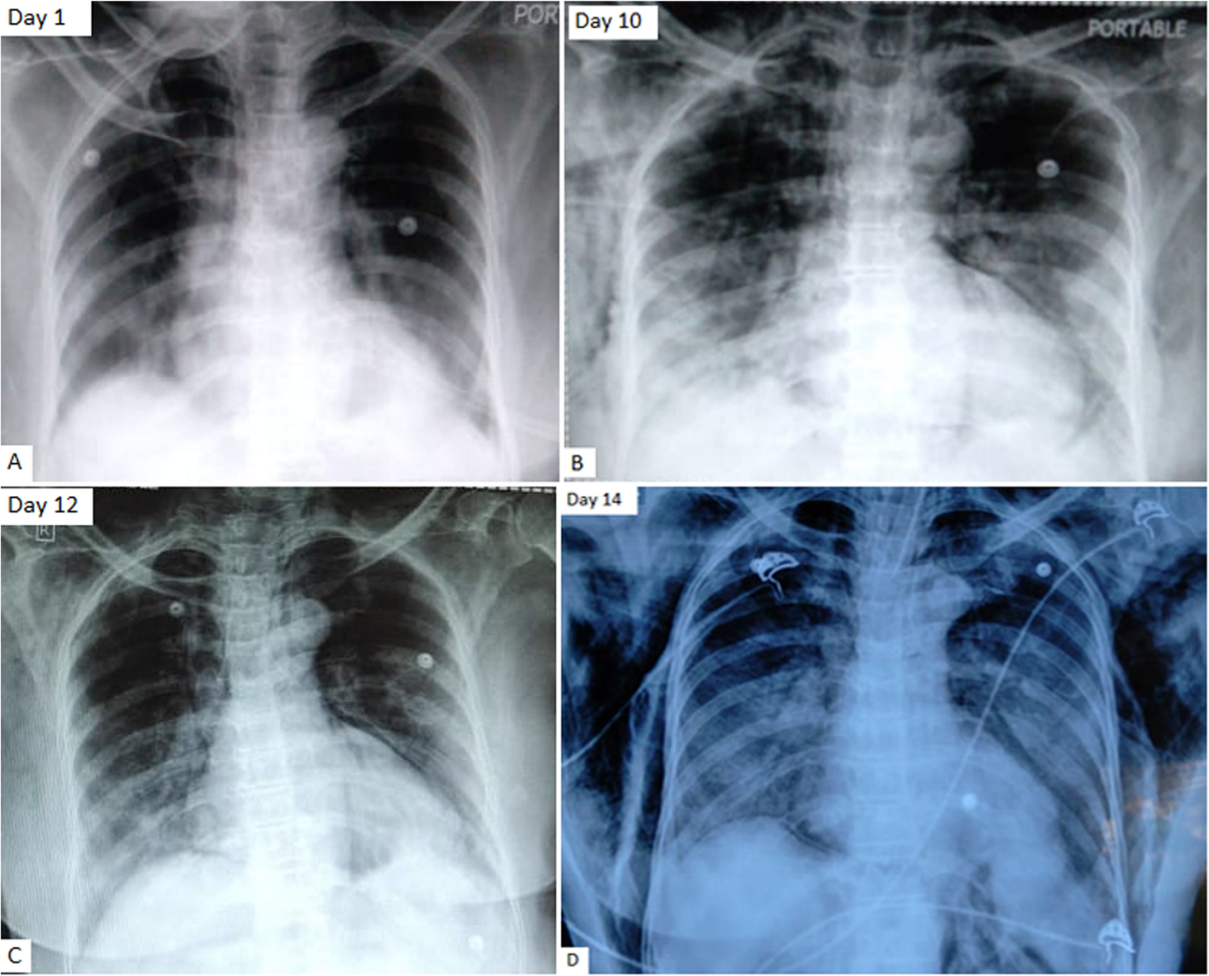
A Day 1 chest X-ray antero-posterior view showing bilateral pneumonia. B Day 10 chest X-ray showing subcutaneous emphysema and pneumopericardium. C Day 12 chest X-ray partial resolution of subcutaneous emphysema. D Day 14 chest X-ray showing recurrence of subcutaneous emphysema with an increase in lung shadows
